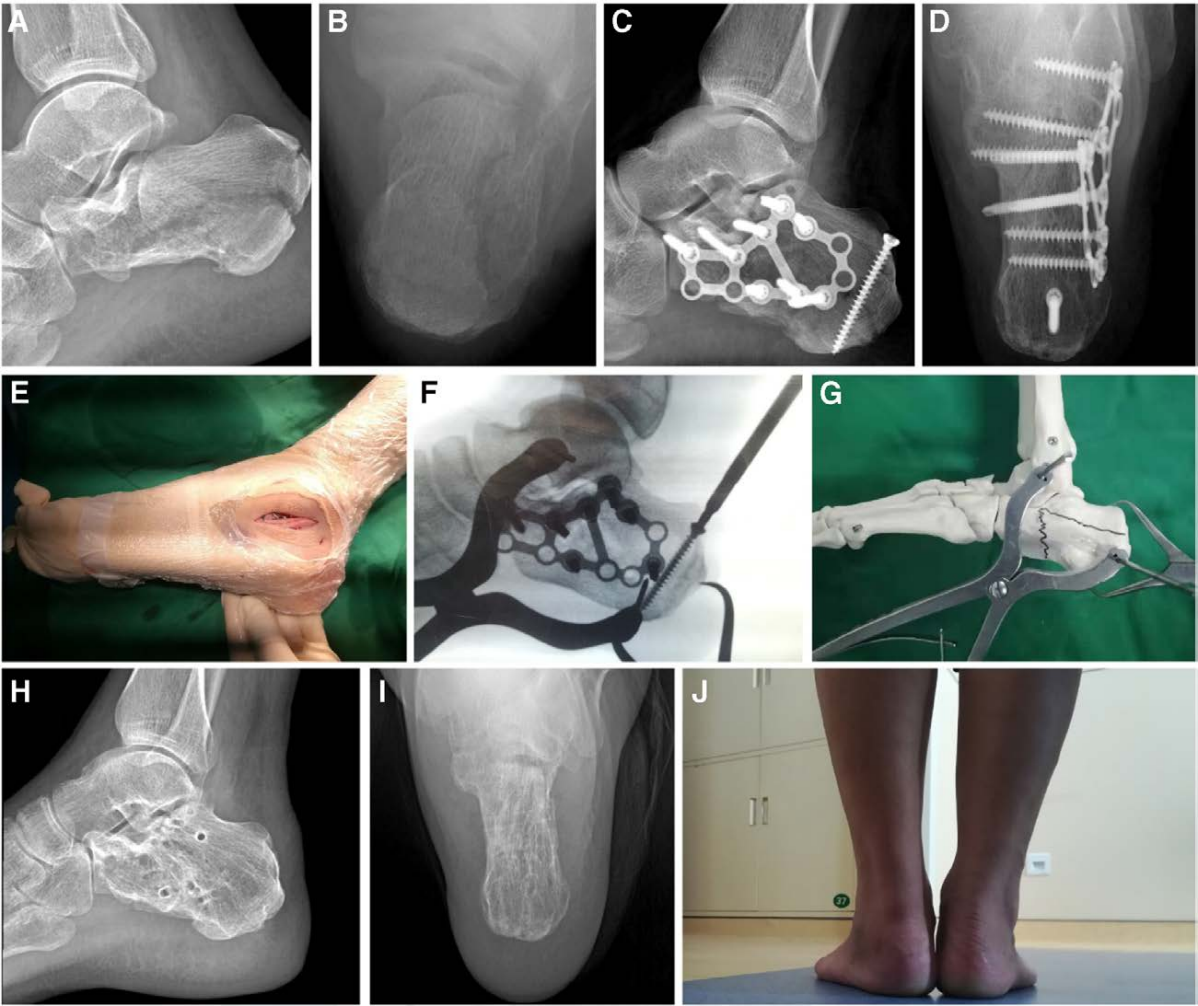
A 47-year-old male patient fell from a height of 3 meters. (A and B) Preoperative radiograph showing left calcaneal fracture (Sanders type IIB) with unnormal Böhler angle (–2°), height (82.7 mm), length (89.4 mm), and width (52.5 mm). (C and D) The postoperative radiograph showing Böhler angle (37°), height (91.6 mm), length (87.3 mm), and width (36.1 mm) are restored. (E, F and G) The protocol of Steinmann pin retractor-assisted reduction with circular plate fixation via sinus tarsi approach was used. (H and I) At the last follow-up, the radiological parameters were well maintained. (J) The patient had a normal alignment of the calcaneus and stable plantigrade foot, and the AOFAS score was 100. AOFAS = American Orthopedic Foot and Ankle Society.
